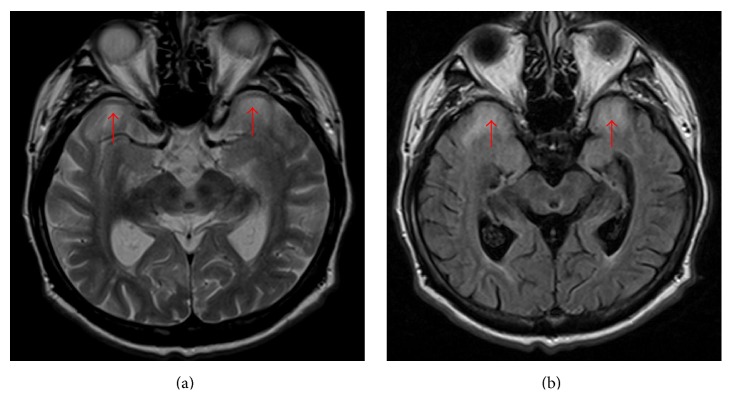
Brain MRI. Anterior temporal lobe hyperintensities on T2-weighted (a) and FLAIR (b) images.
